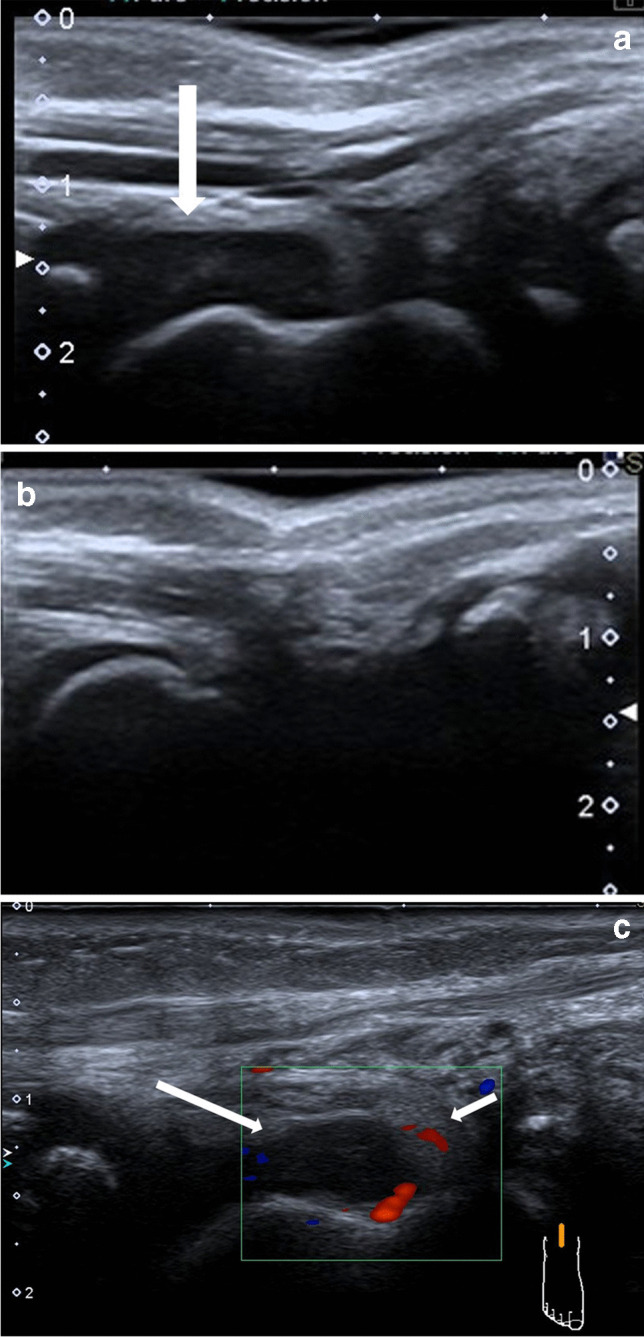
Ankle ultrasound of a 2-year-old girl with juvenile idiopathic arthritis (HLA B27 negative, anti-nuclear antibodies positive). a, b Sagittal projection of right (a) and left (b) ankle with effusion in the right side (*arrow*); (c) Sagittal power Doppler study of right ankle with effusion (*long arrow*), and thickened and hyperemic synovium (*short arrow*)—signs of synovitis
